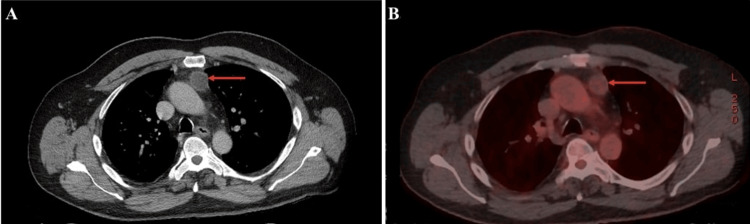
Preoperative (a) CT and (b) PET-CT showing a 2.9 cm low attenuating nodule with some peripheral enhancement and mild adjacent fat stranding within the anterior mediastinal fat just adjacent the ascending aorta
